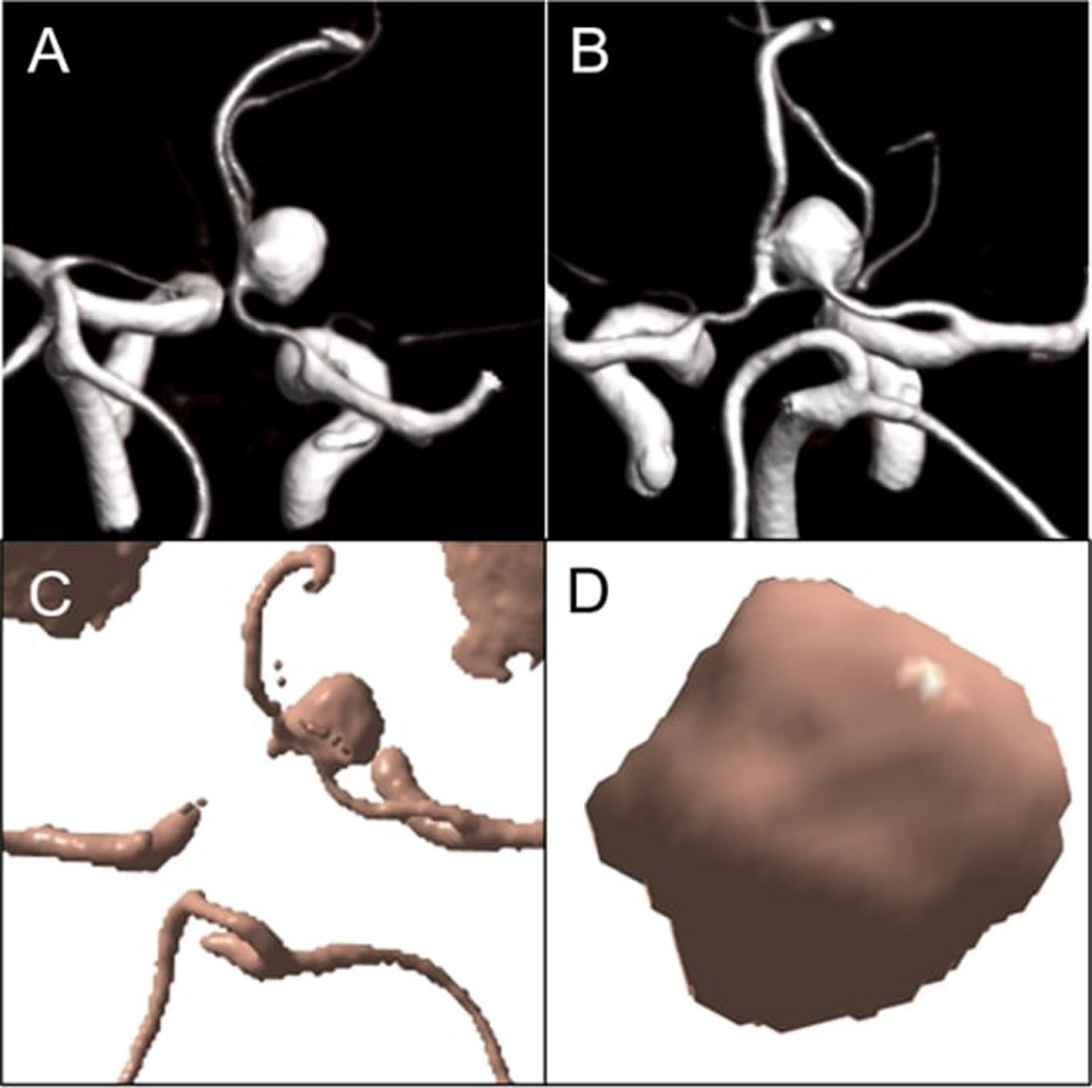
CT-angiography reconstructions of the anterior communicating artery aneurysm (A, B). Post-processing of the 4D-CTA scan after application of a Hounsfield Unit threshold (C) and after application of masks (D). Images A and B obtained using Syngo.Via VB30 software (Siemens Healthineers, Erlangen, Germany) and images C and D using MATLAB 2015b software (MathWorks, Natick, MA).
